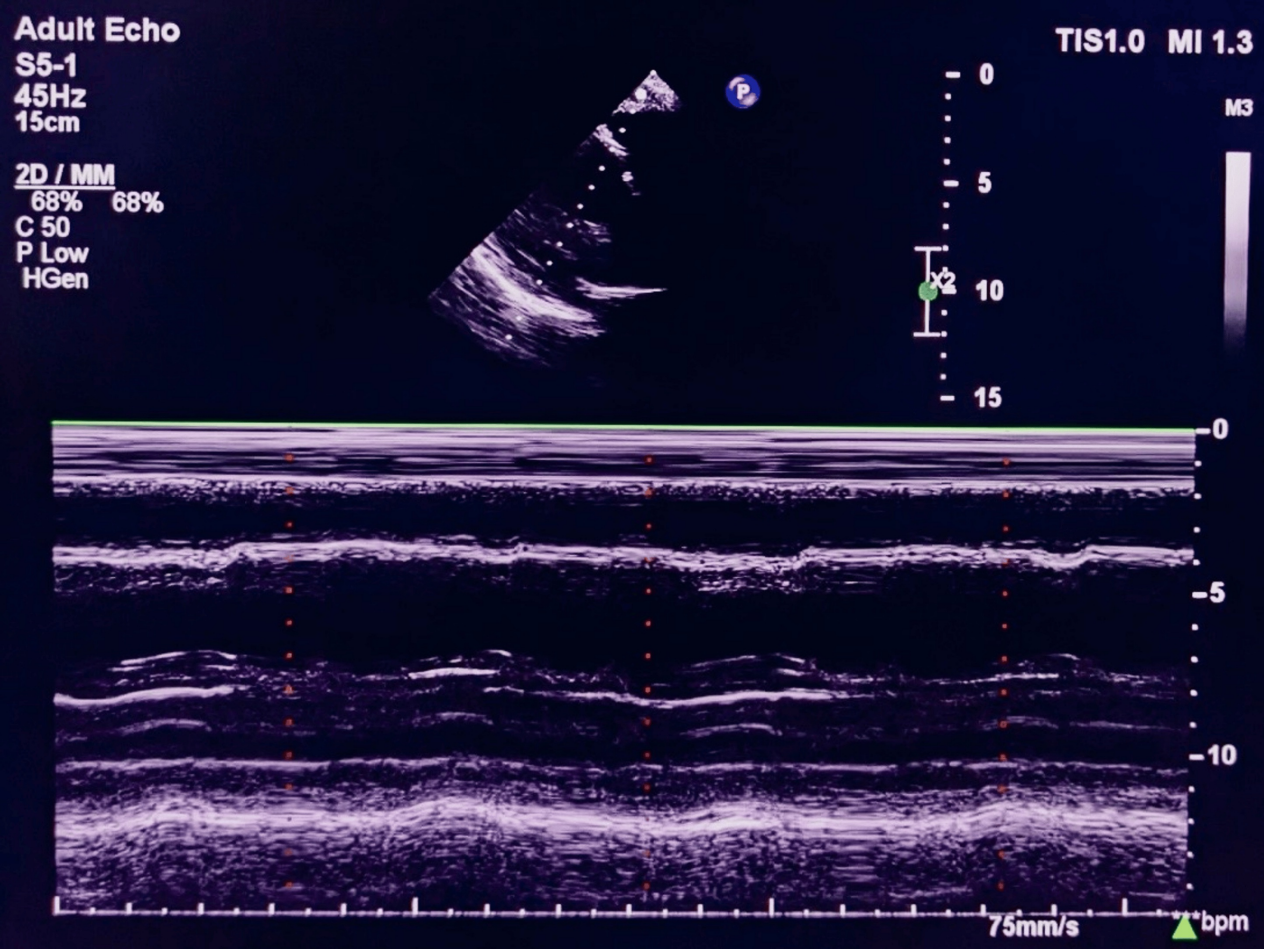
Transthoracic M-mode echocardiogram shows increased end-diastolic and end-systolic left ventricular diameters with global hypokinesia (HFrEF-35%) HFrEF: Heart failure with reduced ejection fraction
